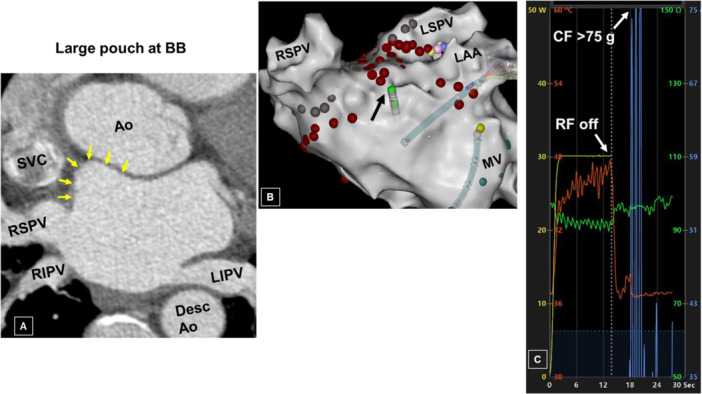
Pouch at the BB region. (A) A computed tomographic axial reconstruction showing a large pouch (arrows) at the region of Bachmann's bundle (BB). Ao, aorta. (B) A 3D anatomic map of the LA during RFA from another patient. As the catheter (black arrow) was deflected anteriorly, it suddenly dislodged, prompting immediate discontinuation of energy delivery (see next). (C) Note the very high contact forces (> 75 g) encountered upon catheter dislodgement into the pouch.
